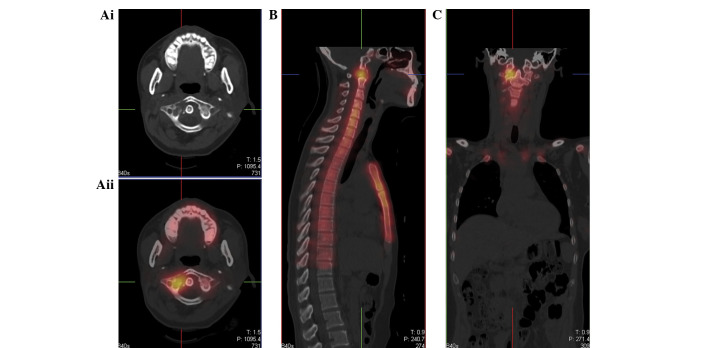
(Ai) Axial CT scan showing osteolytic destruction of the C1 vertebra. Radionuclide bone scan after injection of 24 mCi of ^99m^TC-MDP showing (Aii) the axial view of the cervical spine, (B) sagittal image of the cervical spine and (C) coronal image of the cervical spine. Abnormal activity was present in the C1 region (yellow region in Aii, B and C). These areas of increased radiopharmaceutical uptake represent metastatic skeletal deposits. CT, computed tomography.
